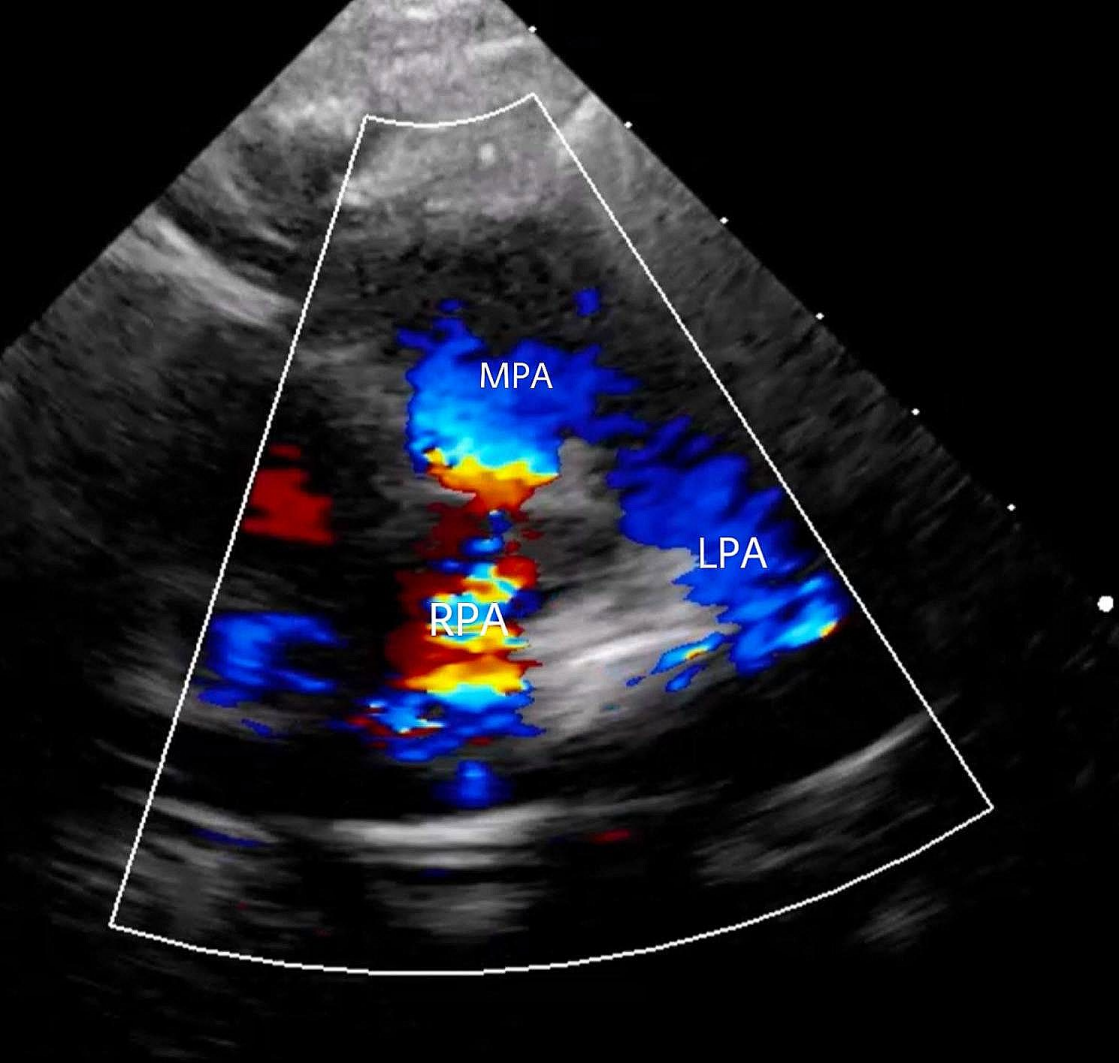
Postoperative transthoracic echocardiography. Postoperative transthoracic echocardiography (short axis view): demonstrating the anatomy of the pulmonary arteries with patent branch right and left pulmonary arteries. With the left pulmonary artery having a peak pressure gradient of 20 mmHg, with mild acceleration of flow across the right pulmonary artery with peak pressure gradient of 20 mmHg *(MPA: main pulmonary artery, RPA: right pulmonary artery, LPA: left pulmonary artery)* (see supplementary video 1)
